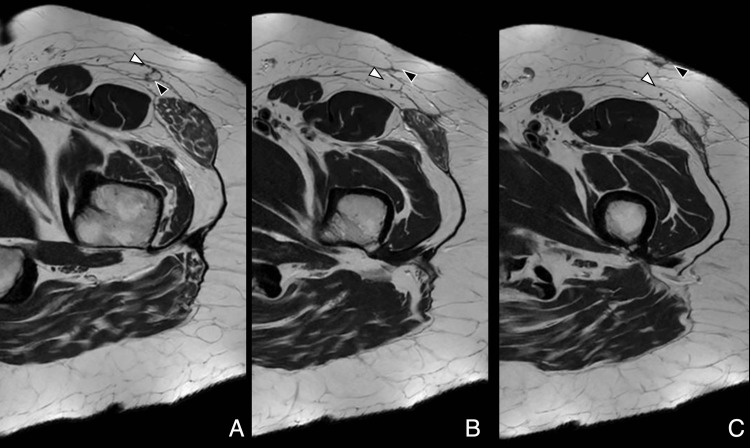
Normal anteromedial portal scar adjacent to LFCN Axial T2-weighted images, proximal to distal, showing linear scar tissue (black arrowhead) along the anteromedial portal tract adjacent to the intact LFCN (white arrowhead). No disruption noted. Patient was asymptomatic. Image Credit: Juan Pablo Muñoz, corresponding author LFCN: lateral femoral cutaneous nerve
